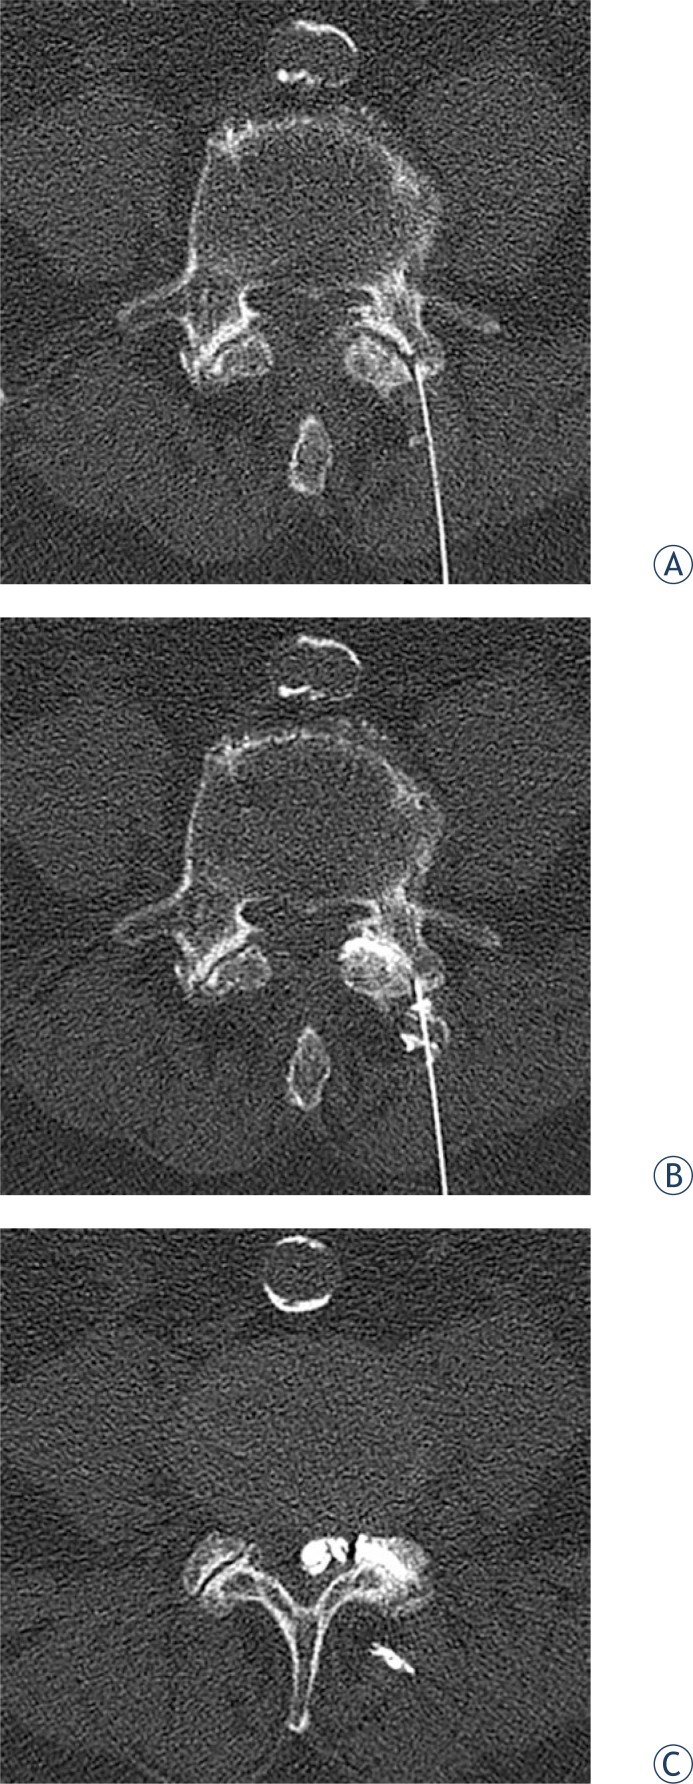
Intraprocedural CT in an axial plane at the level of the L4–L5 lumbar segment. A 22G needle that has entered a posterior part of a target facet joint can be seen (A). Iodine contrast media instilled through the needle has filled the facet joint, confirming the needle tip position inside the joint (B). Continuous contrast instillation resulted in intraspinal contrast opacification of the cyst (confirming the diagnosis of intraspinal synovial cyst) (C). A loss of resistance was felt at the time of cyst rupture. The procedure was terminated at that point.
